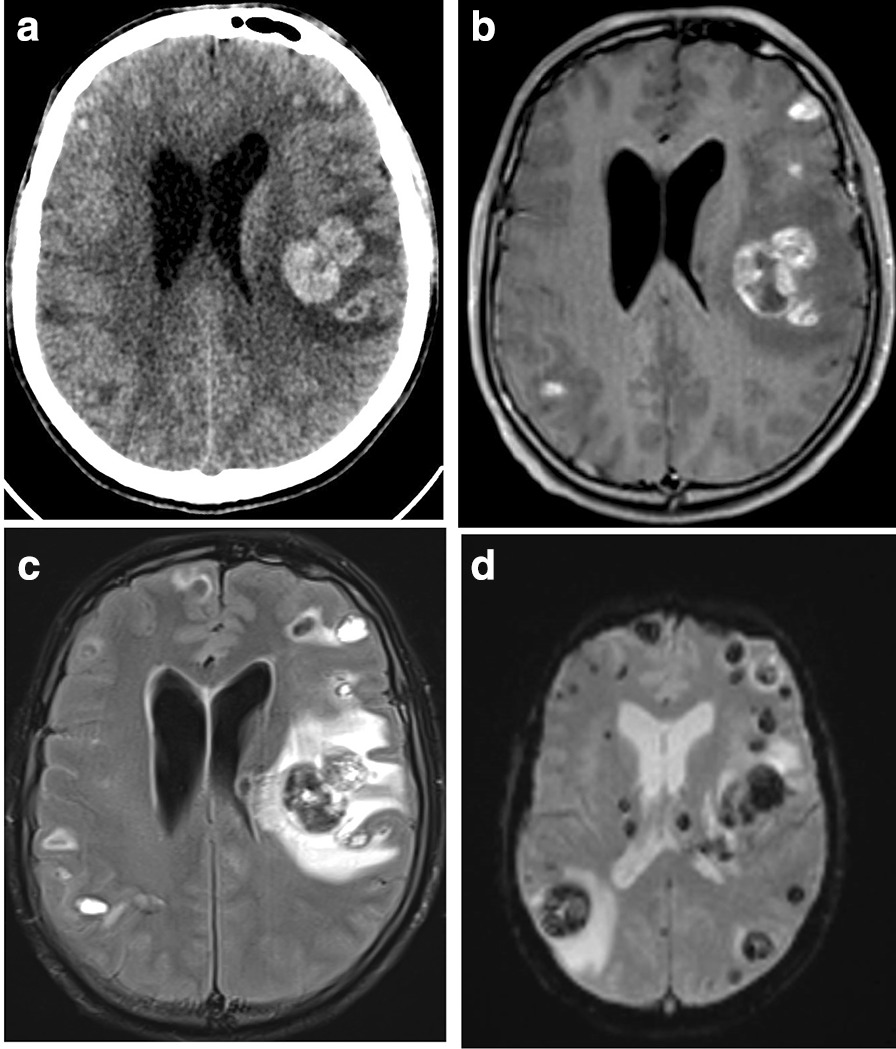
Axial nonenhanced computed tomography (NECT) (A) showing multiple hyperdense lesions. The hemorrhagic lesions were very heterogeneous on T1- (B), T2-FLAIR (C), and perfusion (D)-weighted MRI images. The main lesion in the left deep white matter is surrounded by edema, with a mass effect on the ventricle
